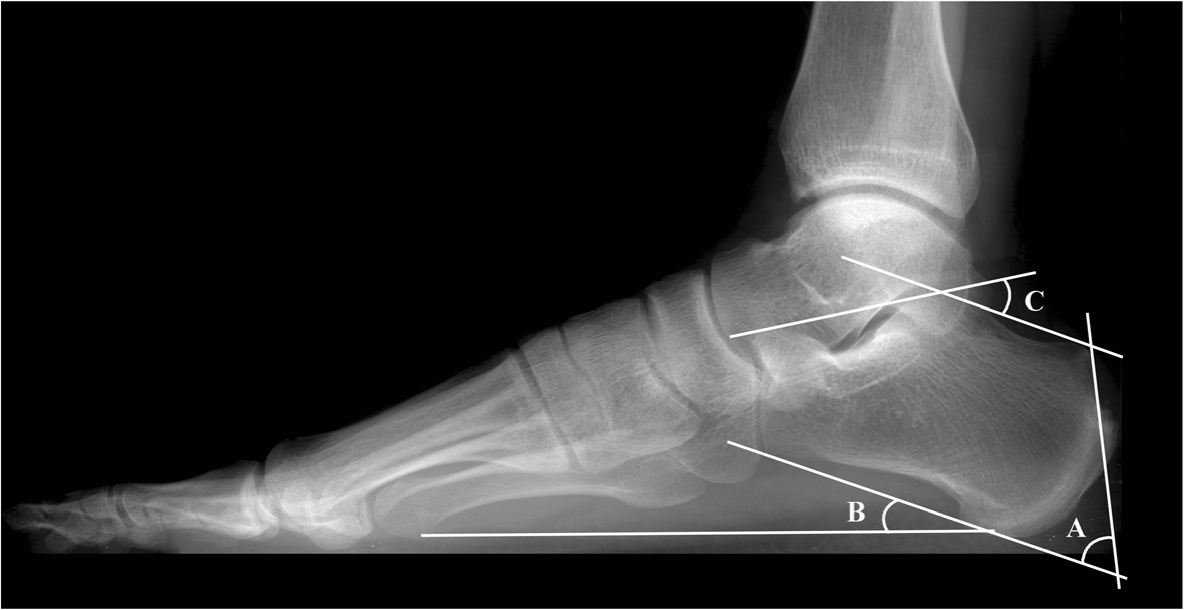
Radiographic indices evaluated using standing lateral foot radiograph. a Fowler-Philip angle. b Calcaneal pitch angle. c Bohler’s angle
